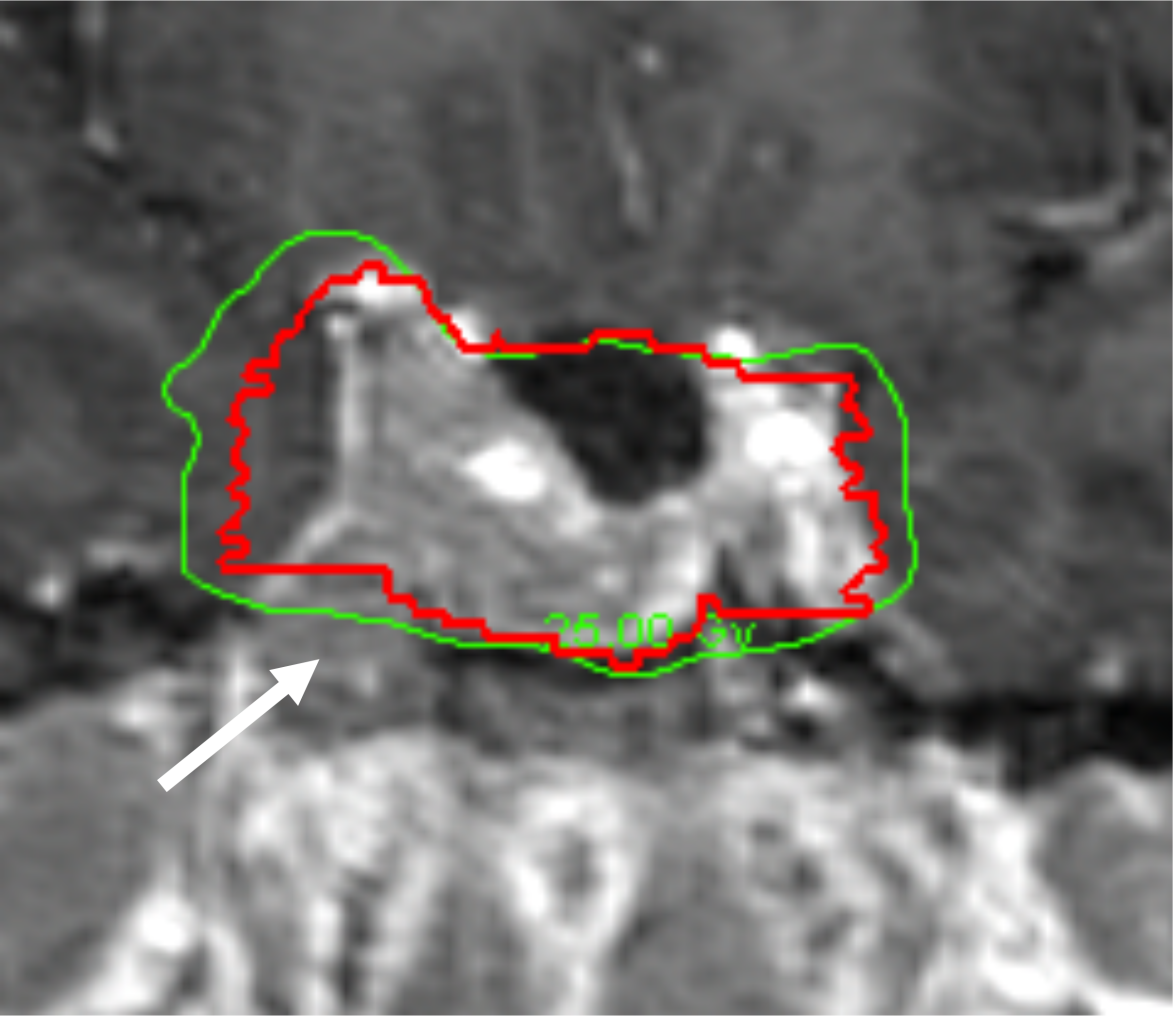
Example of a radiographically confirmed out-of-field failure in a patient with a large tumor (>20 cc) treated with a lower dose (25 Gy). Red: gross tumor volume (GTV) at the time of treatment. Green: isodose line that indicates the prescribed dose of 25 Gy. See tumor progression inferior to the prescribed dose of 25 Gy (white arrow).
